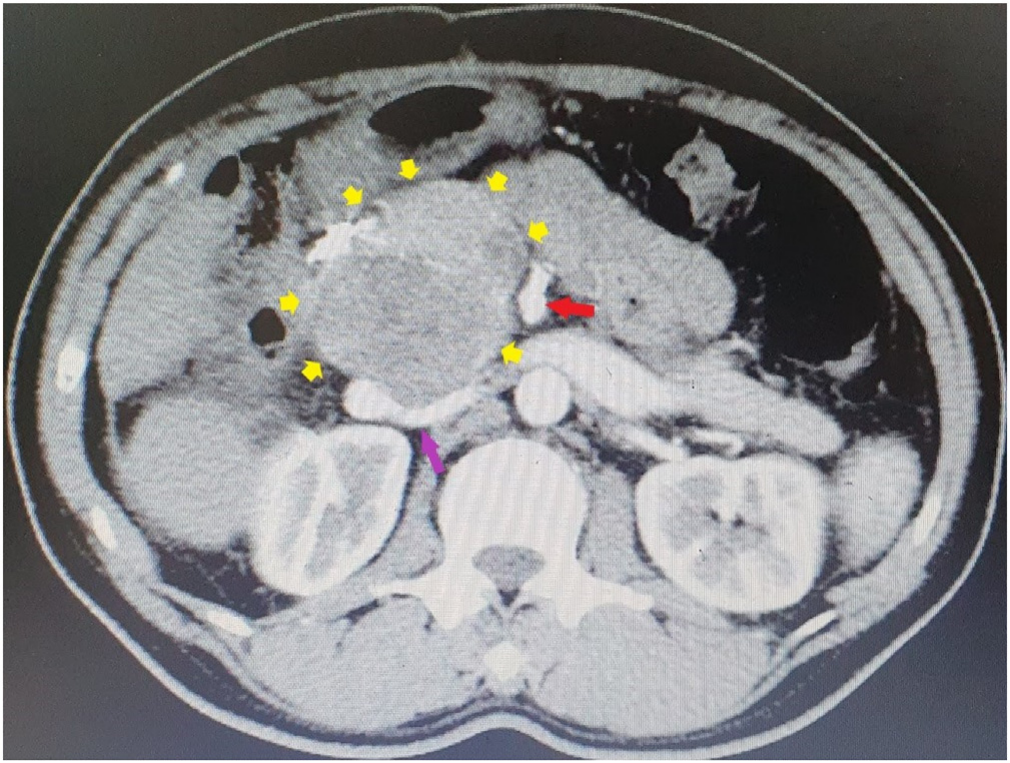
Computed tomography image of posterior large lymph node (yellow arrow). Upper mesenteric artery (red arrow), right renal artery (pink arrow). The lymph node metastatic close to the S1r position with size of 34 × 60 mm. (For interpretation of the references to colour in this figure legend, the reader is referred to the web version of this article.)
